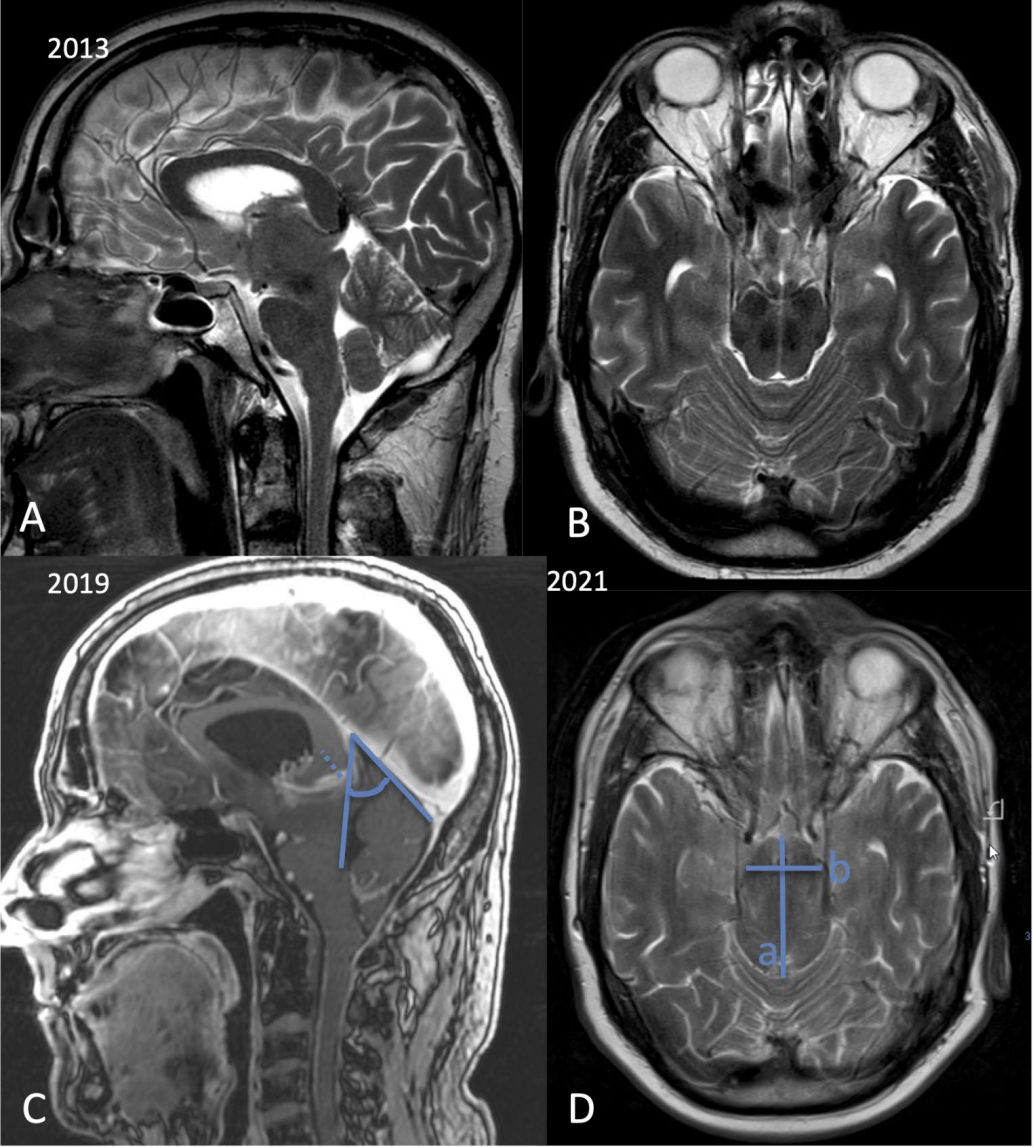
A 57-year-old man presented with apathy, loss of interest, gait ataxia, and tremor. Over at least 6 years symptoms had progressed so that he was only sitting looking television the whole day. Sagittal (A) T2-weighted and contrast enhanced MPRAGE images (C) show a small angle between the vein of Galen and the straight sinus (C: angle). The vein of Galen is elongated as indicated by the distance from the confluence of the internal cerebral veins and the exit of the vein of Galen in the straight sinus (C: dashed arrow). Axial T2-weighted images (B, D) show a marked and progressive midbrain elongation indicated by a long a.p. diameter of the midbrain and a higher sag ratio a/b
